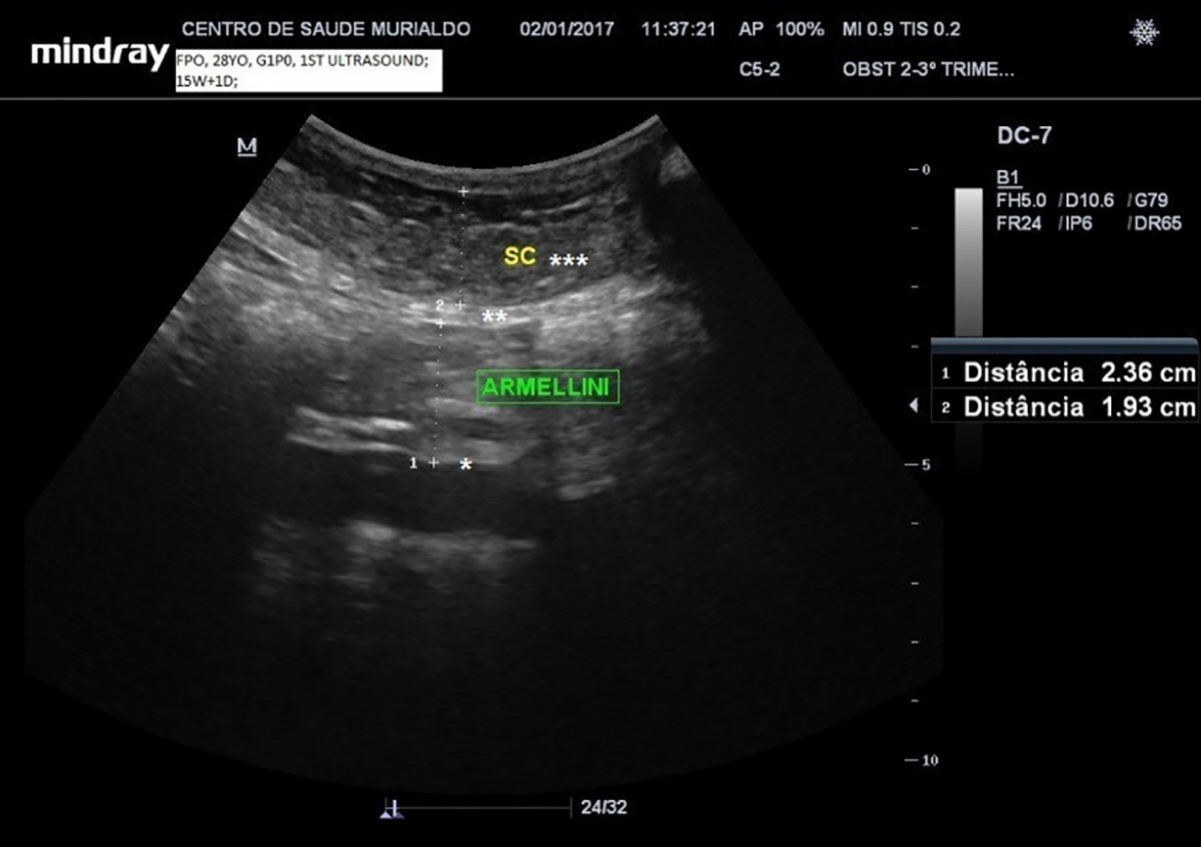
VAT ultrasound measurement according to the *Armellini´s* technique. (*) Abdominal aortic anterior wall. () *Linea* Alba. (*)Subcutaneous fat.
